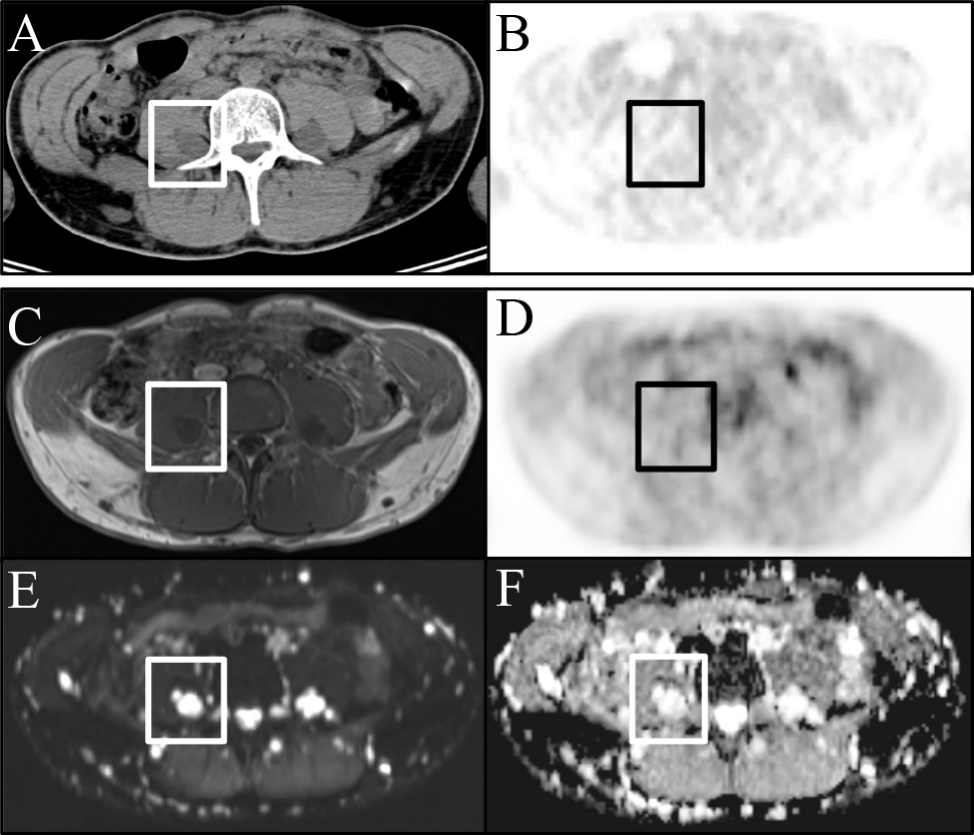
PNST in a 22 -year male patient. (A) CE-CT image and corresponding (B) PET image with no pathological glucose uptake. (C) T1w image and corresponding (D) PET images with also no abnormal focal tracer uptake. (E) DWI (b800) and (F) ADC maps showing restricted diffusion but high signal on the ADC map.
